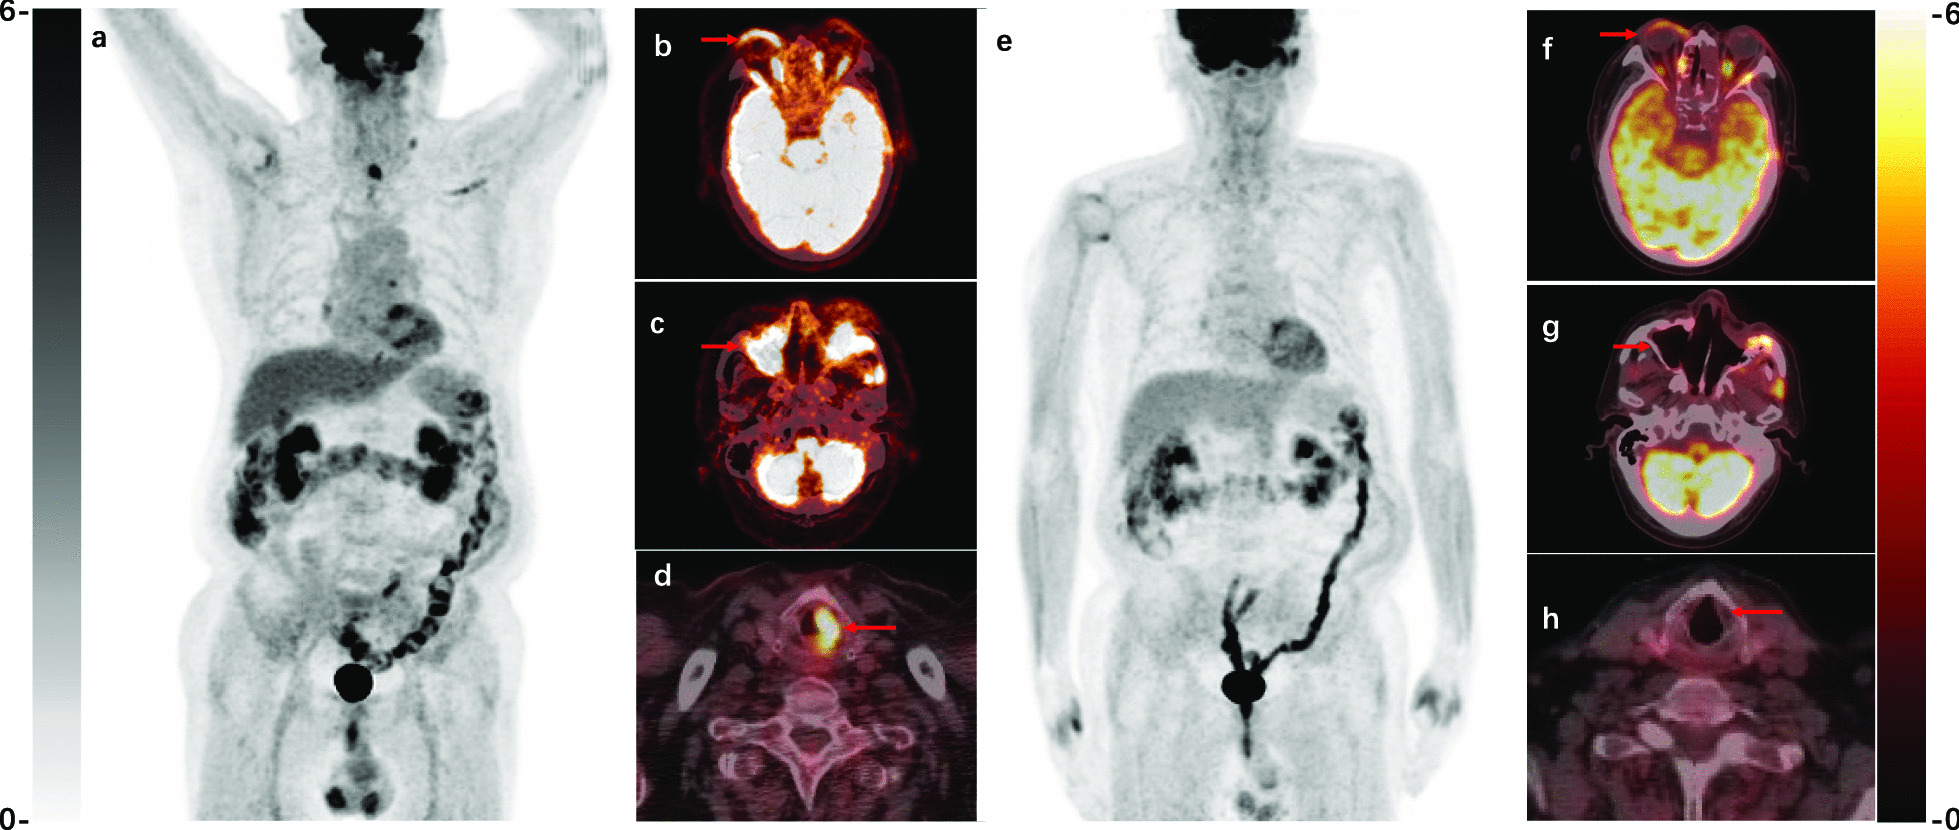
A typical case showing the utility of ^18^F-FDG PET/CT to follow-up of RDD. ^18^F-FDG PET/CT images of RDD with partial response in a 70-year-old man, after 4-month cytarabine. a–d Pretreatment PET/CT images displaying hypermetabolic eyelids (SUVmax 7.4), maxillary sinus (SUVmax 9.3) and left plica vocalis (SUVmax 9.0). e–h Follow-up scans showing lesion resolution with metabolic uptake and volume decrease: eyelids (SUVmax 4.3), maxillary sinus (SUVmax 5.7), left plica vocalis lesions disappeared
